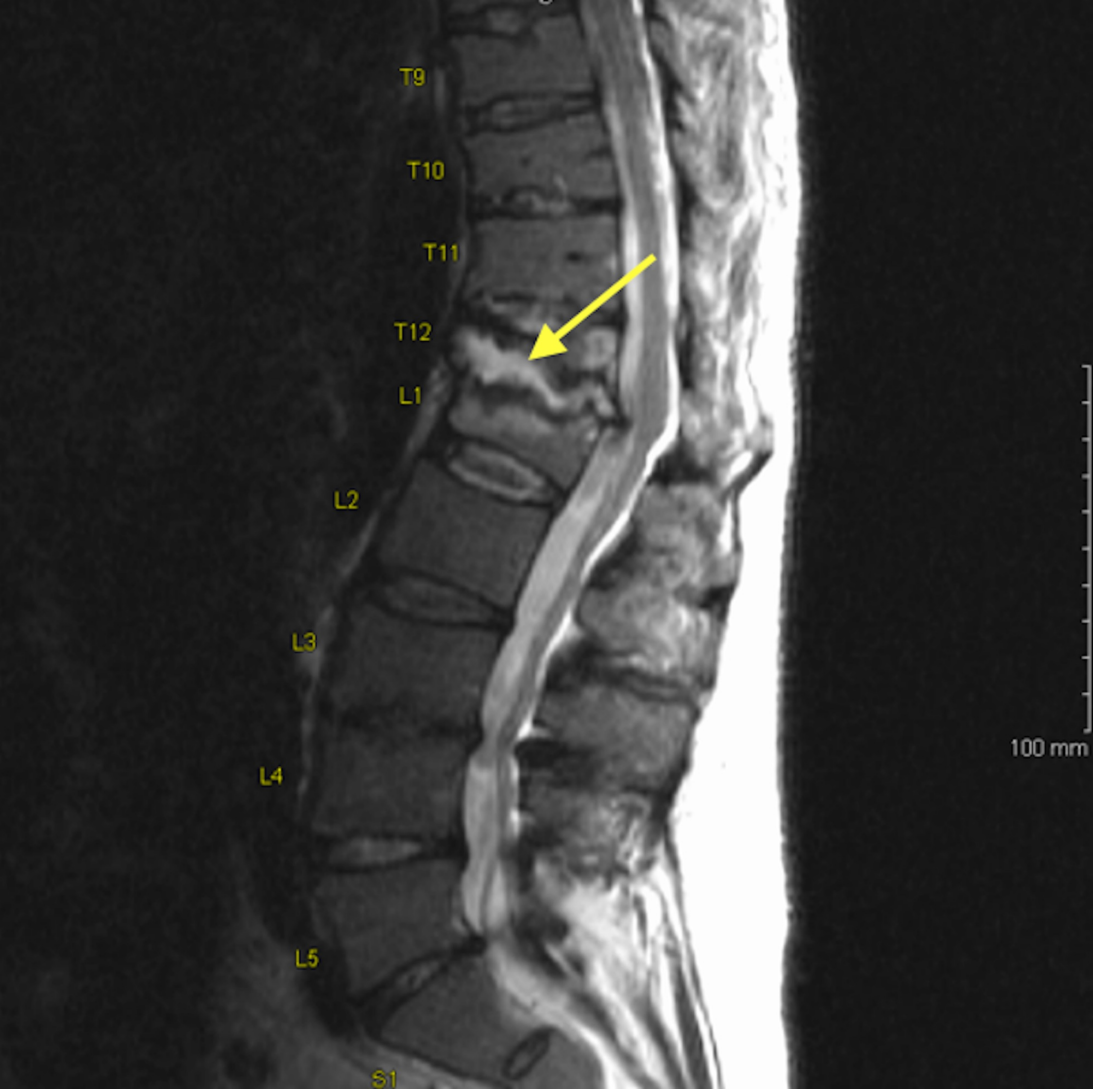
MRI of lumbar spine showing degenerative changes at T12/L1 level consistent with spondylodiscitis with vertebral osteomyelitis (arrow).
